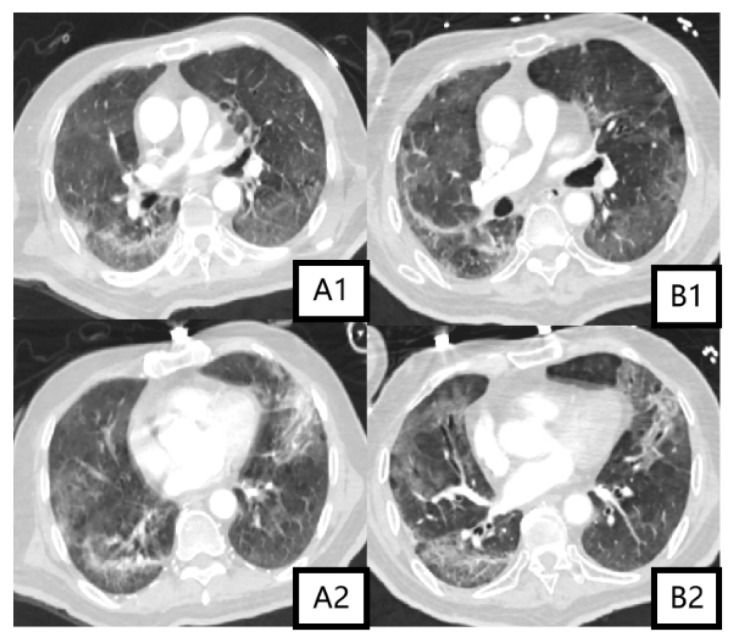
On the 10th hospital day, chest CT revealed multiple patchy ground-glass opacities in both lungs, with some irregular consolidation (30 November 2023; (A1,A2)). On the 17th day after admission, chest CT showed that ground-glass opacities and consolidation were partially absorbed in both lungs (7 December 2023; (B1,B2)).
